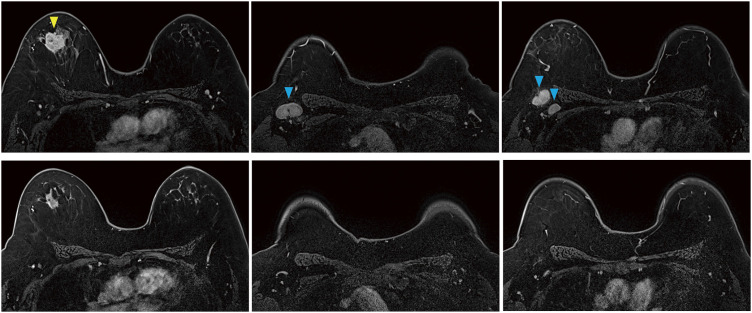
Breast MRI images before (upper) and after (lower) NAC (fat suppression T1-weighted early phase). The tumor and lymph nodes with strong enhancements (yellow and blue arrowheads) disappeared after NAC. NAC, neoadjuvant chemotherapy
